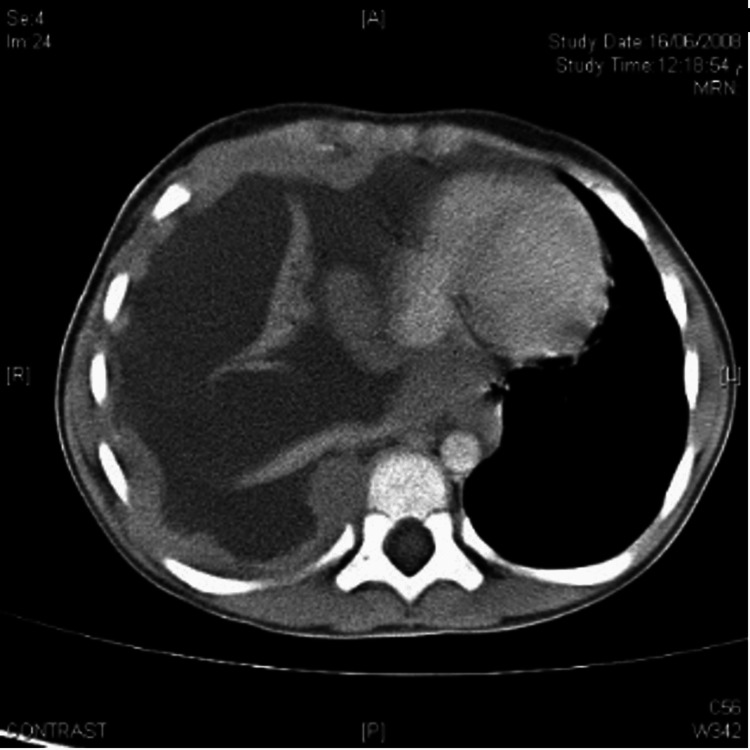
Computed tomography (CT) scan, axial view, mediastinal lung window shows a marked right-sided pleural effusion, collapsed lung, thick pleural service, shift of mediastinum to the left side.
